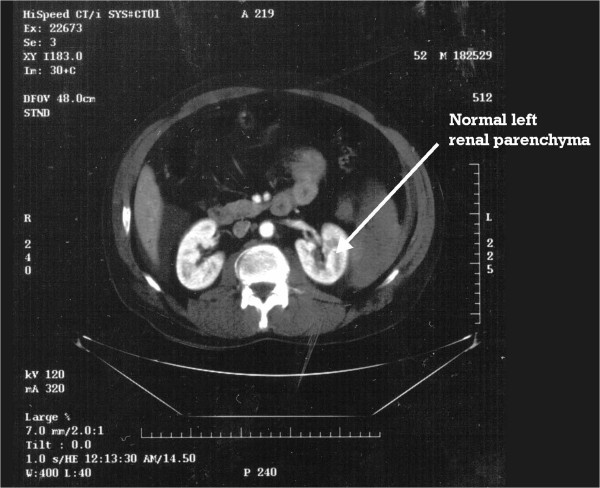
CT scan: the left kidney noted no extravasation or parenchymal injury but with a fractured calculus.
